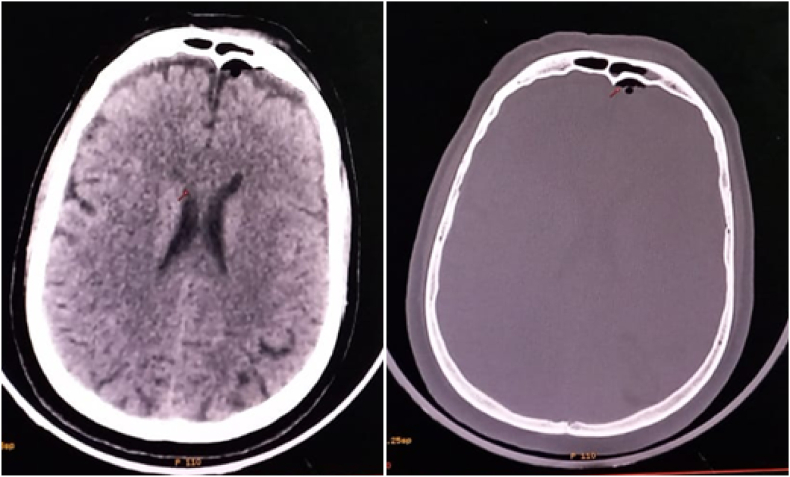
Brain computed tomography, showing frontal pneumocephalus and meningeal hemorrhage.
